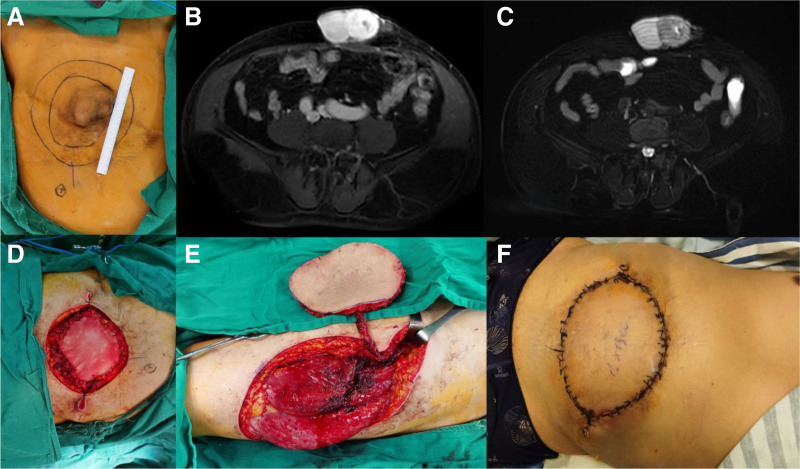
Clinical presentation and MRI findings of case 3. (A) DFSP lesion located in the abdominal region; (B–C) Magnetic resonance imaging before surgery; (D) tumor and adjacent abdominal muscles and peritoneum were resected, and polypropylene mesh was used to cover the defect; (E) free anterolateral thigh flap was harvested; (F) Immediate post-operative picture with the flap sutured to the abdominal wall defect.
